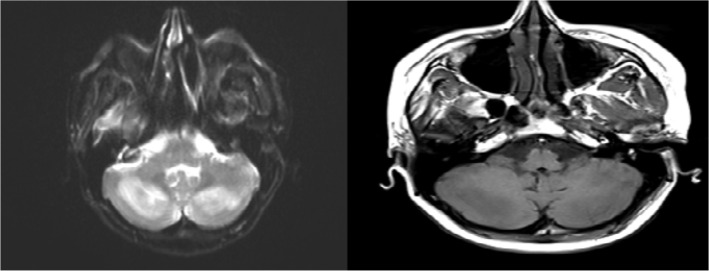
T2/FLAIR image of brain MRI. A 25-year-old female with systemic lupus erythematosus, presenting seizures after hypertension. Hyperintense signal changes in T2/FLAIR sequences in the left posterior frontal lobe, bilateral occipital, posterior parietal lobes, basal ganglia, and cerebellar hemispheres.
